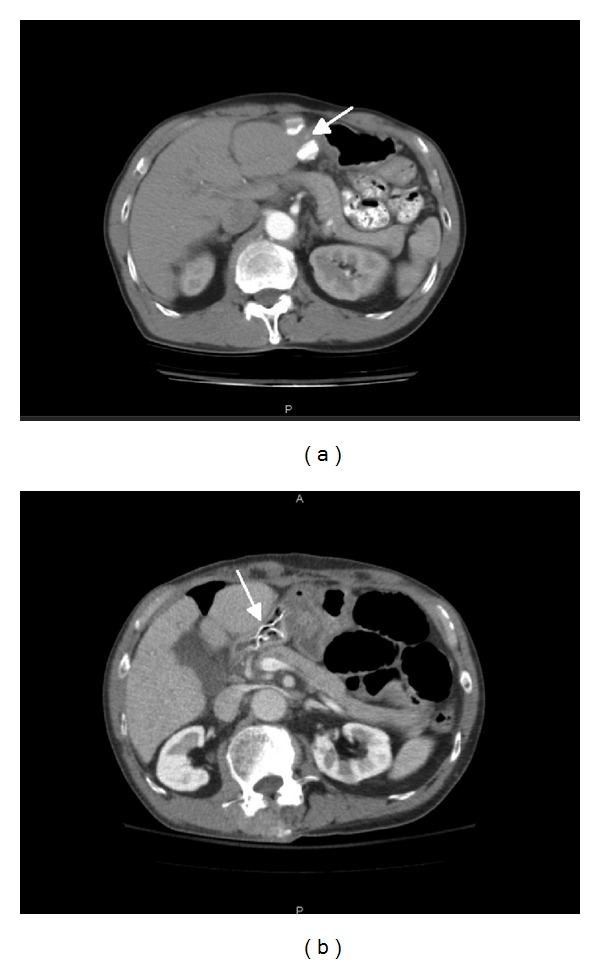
(a) Left, computed tomography scan 7 years after resection, showing the mesh (arrow) extraluminal to the stomach and duodenum. (b) Right, the mesh (arrow) migrated intraluminally 2 years later.
